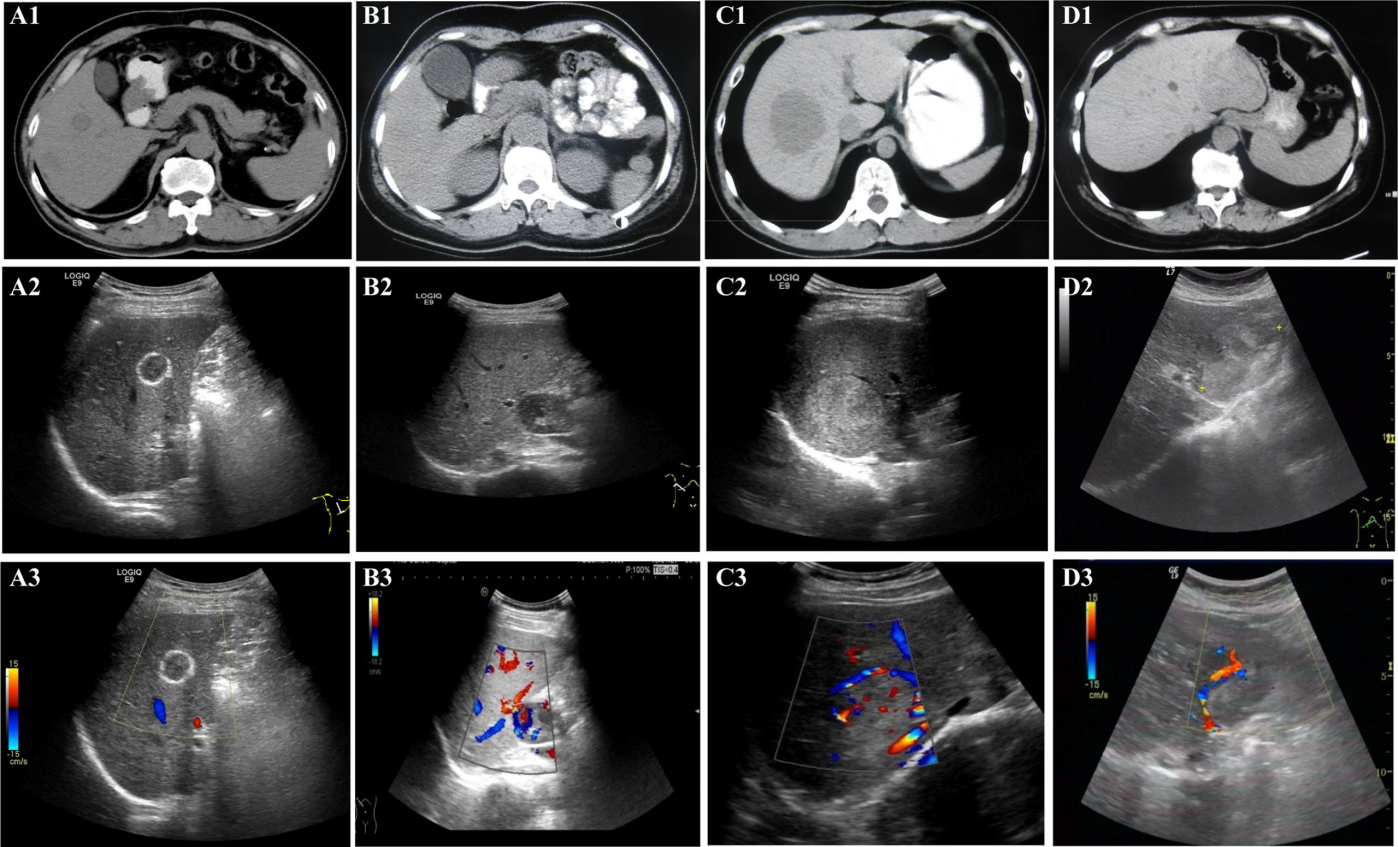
Non-enhanced imaging (CT, US, and CDFI) appearance. All patients underwent non-enhanced imaging examination, including CT, US, and CDFI. A1 (CT), A2 (US), and A3 (CDFI) are images of lesions in the patient 10. B1 (CT), B2 (US), and B3 (CDFI) are images of lesions in the patient 2. C1 (CT), C2 (US), and C3 (CDFI) are images of lesions in the patient 1. D1 (CT), D2 (US), and D3 (CDFI) are images of lesions in patient 4. For CT, (A1, B1, C1, and D1): CT scans showed that all lesions were hypodense. For US, (A2): US showed the lesion with hyper-echoic ring around and iso-echo inside, lateral shadow, clear boundary and regular shape. (B2): US showed hypoechoic lesions with clear boundary and regular shape; (C2): US showed hyperechoic lesion with clear boundary and regular shape; (D2): US showed that the lesion were heterogeneously hyper-echoic, unclear boundary and irregular shape. For CDFI, (A3): CDFI showed no obvious blood flow signal inside and around the lesion; (B3, C3, D3): CDFI showed that there were large blood vessels and blood flow signals inside the lesion.
